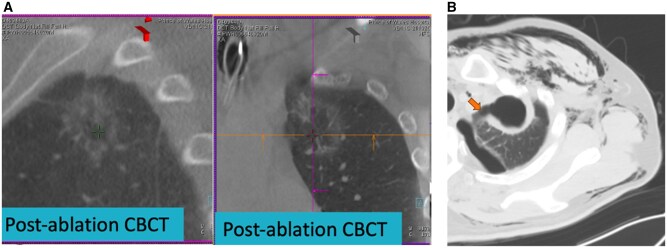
Patient B Intraoperative CBCT and postoperative CT. (A) Intraoperative CBCT showing successful ablation of both lung lesions with adequate margins. No immediate pneumothorax was noted on intraoperative imaging. (B) Postoperative CT of the thorax was performed in view of increased air leakage from the chest drain and increased surgical emphysema. The CT showed an extensive ablation zone with large cavitation and a ruptured ablation zone. Abbreviation: CBCT, cone-beam CT.
